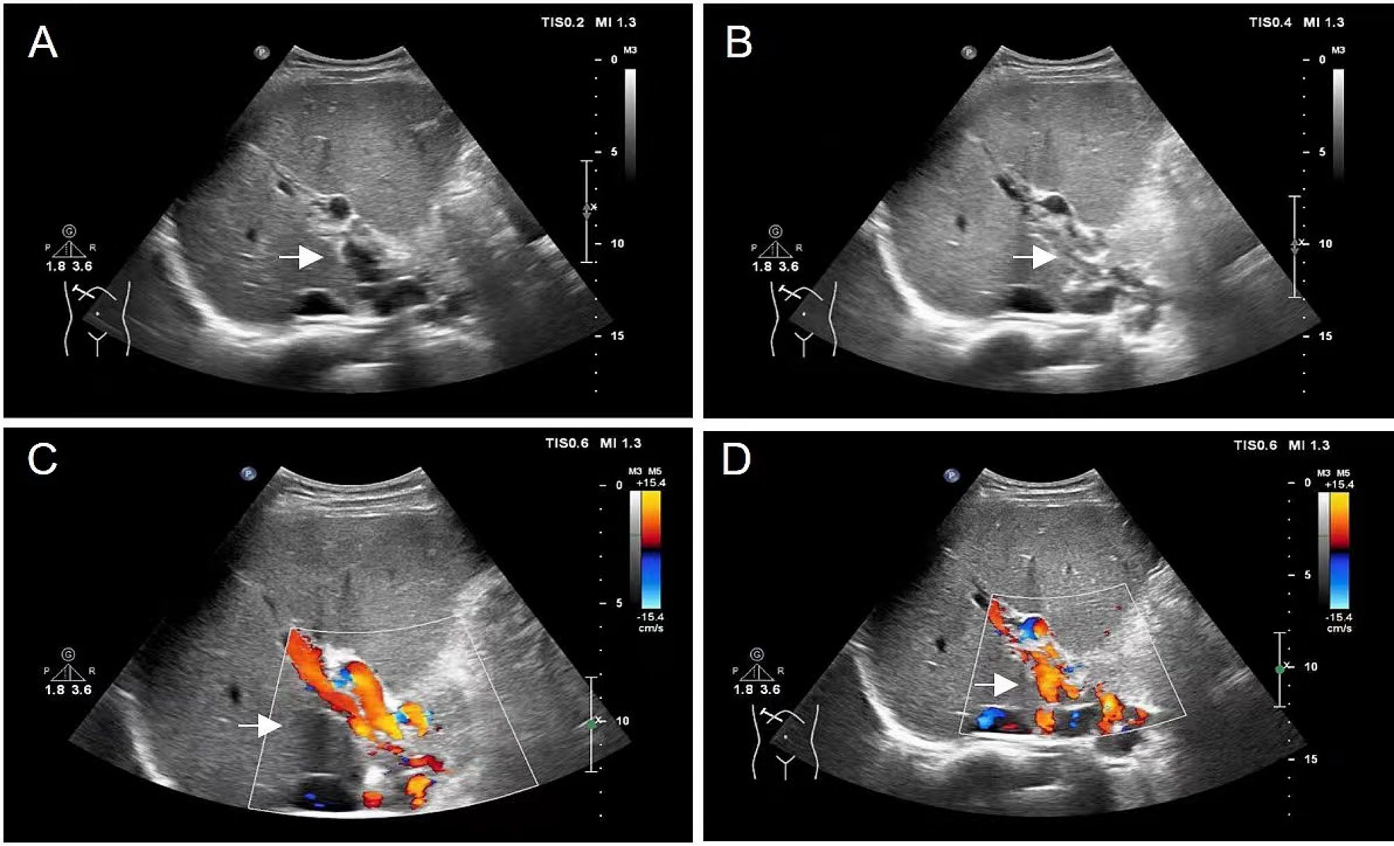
Ultrasonography for the identification of cavernous transformation of the portal vein (CTPV). (A, B) Doppler color flow imaging (DCFI) showing many spot- and slice-style color blood flow signals that are characteristic of CTPV. (C, D) The anechoic area in the first porta hepatis shows dilated vessels
